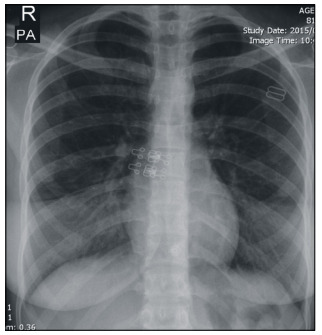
Chest X-ray showing nearly normal lung field.
